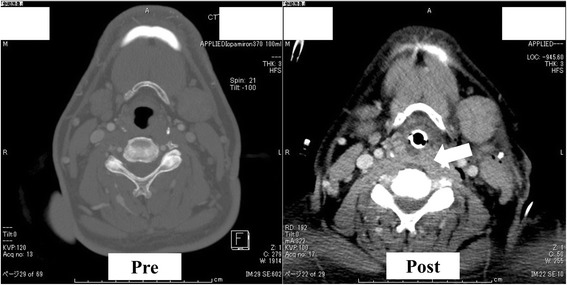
Post-operative image of computed tomography scans shows the edema of the posterior pharyngeal wall was developed compared to the pre-operative state
